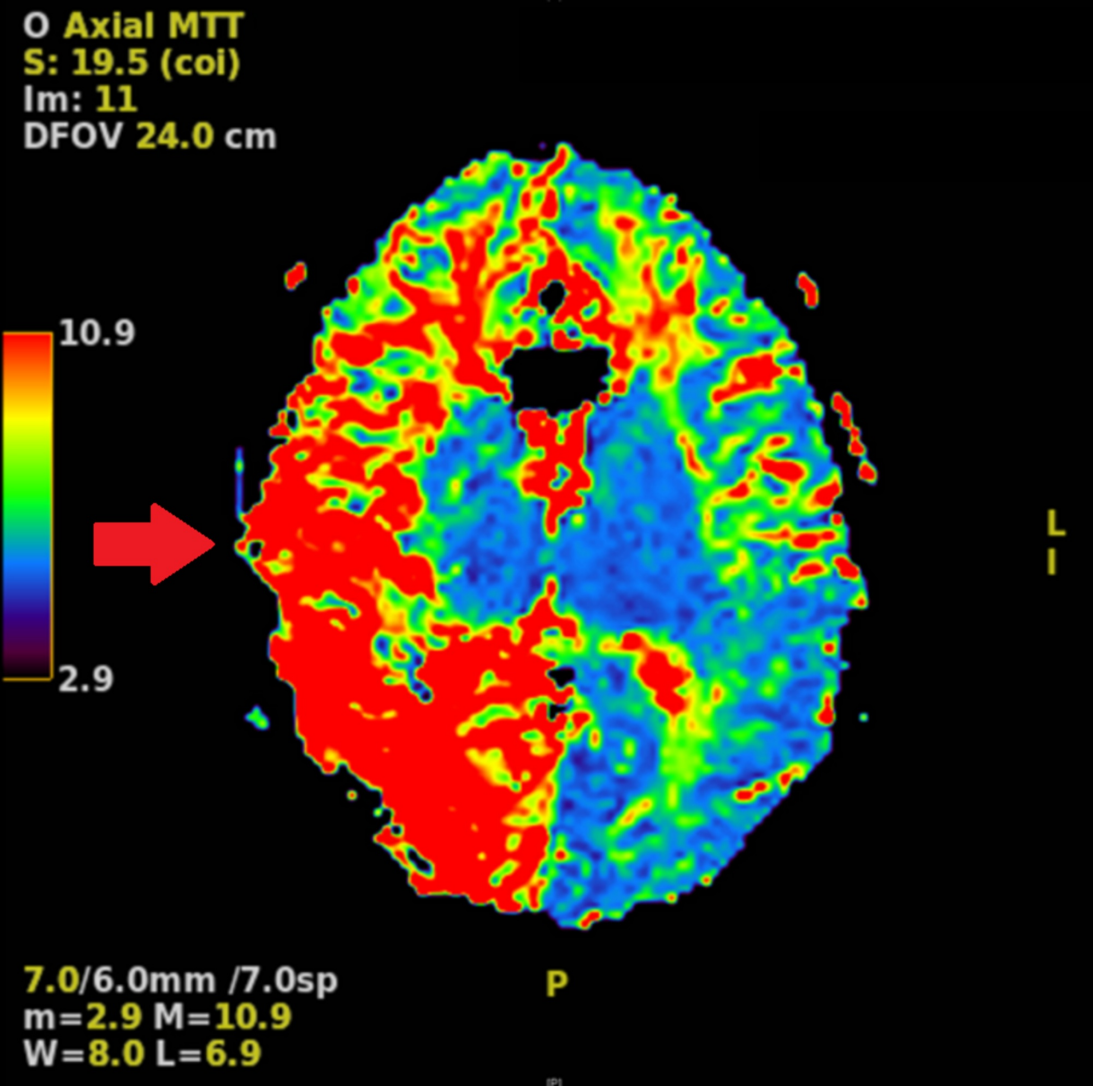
MRI perfusion study demonstrating prolonged mean transit time throughout the right hemisphere relative to the left hemisphere, more predominant posteriorly (red arrow).
